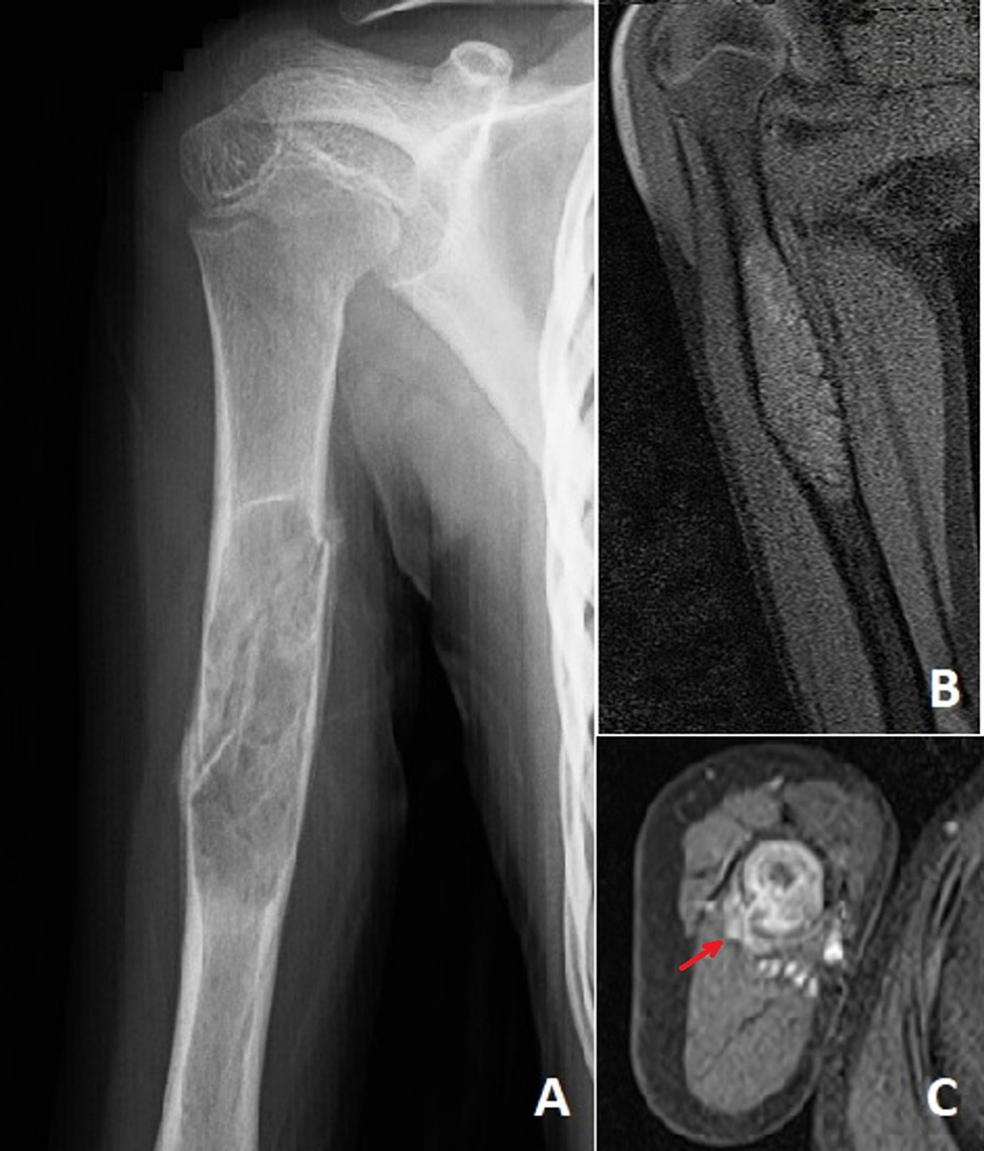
A: Anteroposterior radiography of the right humerus diaphysis with foamy patchy lytic areas of the expansile lesion that caused a fracture. B: T1-weighted coronal MRI showed a medullary lesion without soft tissue component thinning the hypointense expansile cortex. C: T2-weighted axial image showed a heterogeneous hyperintense intramedullary lesion with mild contrast enhancement in the posterolateral parts of the bone (arrow).
